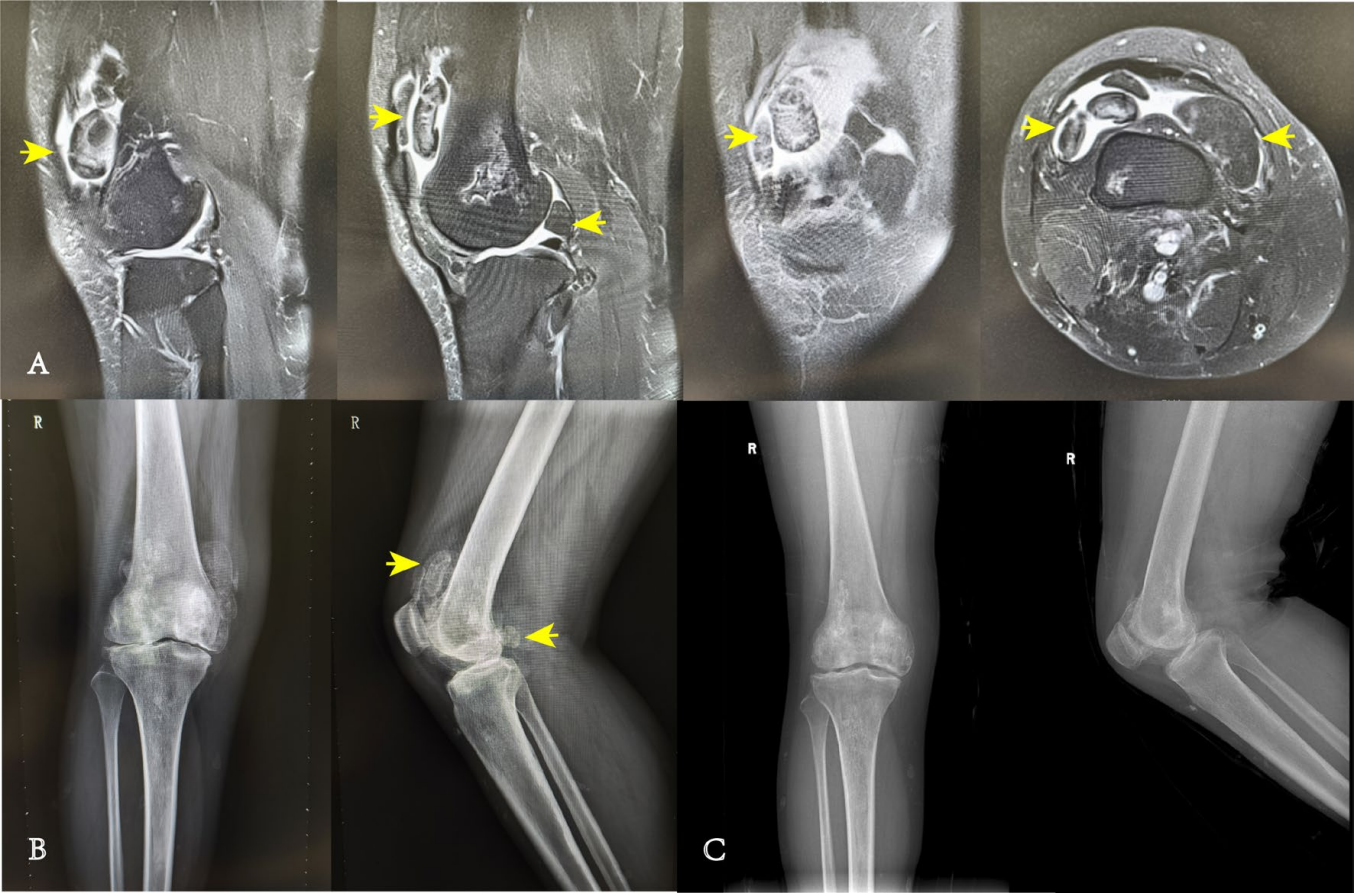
The patient is a 36‐year‐old female who presented with pain and discomfort in her right knee for over 8 years, which had worsened in the past 2 months. Physical examination revealed significant swelling of the right knee, mild tenderness, notable patellar crepitus, and a palpable mass in the suprapatellar pouch. During surgery, extensive hyperemia and thickening of the synovium were observed. Multiple large, smooth synovial chondromatosis nodules of varying sizes were removed from the suprapatellar pouch. Additionally, several broad bean‐sized loose bodies were found in the upper part of the suprapatellar pouch, the posterolateral compartment, and the intercondylar fossa. Pathological examination showed synovial tissue hyperplasia with calcification. Postoperatively, the patient's symptoms improved significantly, and her function recovered well. (A) The patient's MRI shows multiple loose bodies within the knee joint; (B) the patient's X‐ray reveals knee synovial chondromatosis and osteoarthritis; (C) the patient's X‐ray at the last follow‐up. The yellow arrows indicate the loose bodies in the suprapatellar pouch of the knee.
